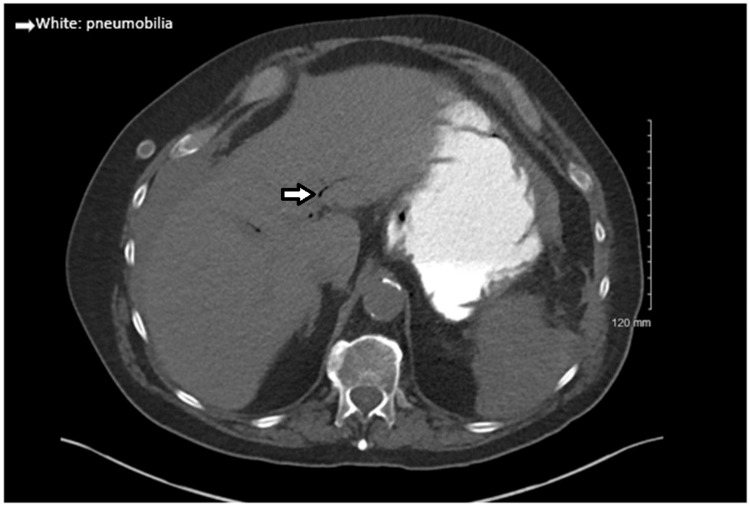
Transverse section of CT abdomen on day three of hospitalization shows pneumobilia without gallstone present (white arrow)
